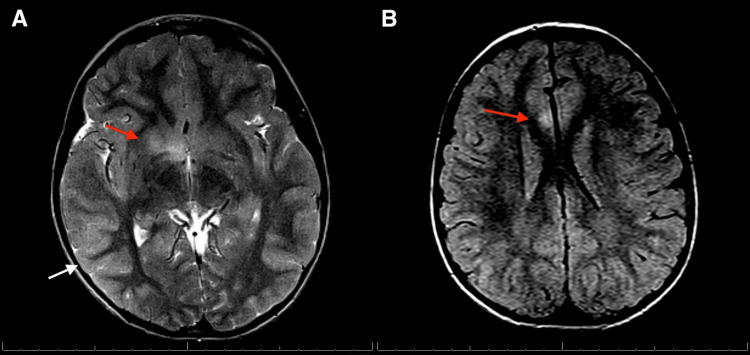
Axial T2-weighted and FLAIR MRI of the brain demonstrates an ill-defined, patchy area of T2/FLAIR hyperintensity involving the inferior margin of the right basal ganglia, located at the medial margin of the right anterior commissure (A, red arrow). Additionally, there is subtle cortical FLAIR hyperintensity involving the bilateral posterior temporal lobes (A, white arrow), extending to the temporo-occipital junctions. A linear strip of FLAIR hyperintensity is also noted along the right cingulate gyrus (B, red arrow). FLAIR: fluid-attenuated inversion recovery.
